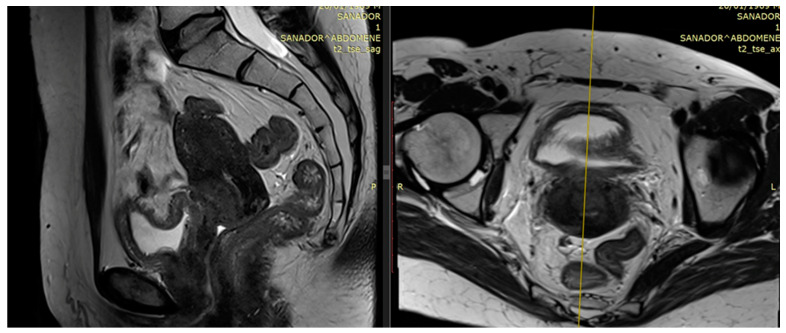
Patient 2 T2-weighted pre-brachytherapy MRI (5th week of CRT): residual tumor and irregular tumor signal extending to parametrium, bladder, and vaginal wall—sagittal view and axial view.
